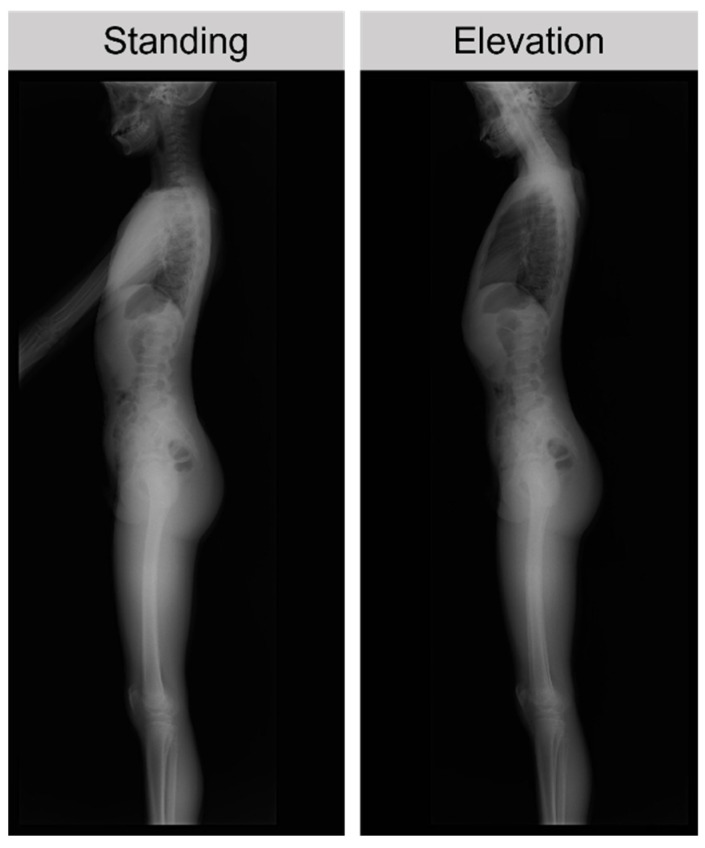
Patient position for X-ray evaluation. The patient was instructed to elevate both upper limbs to the maximum during elevation.
